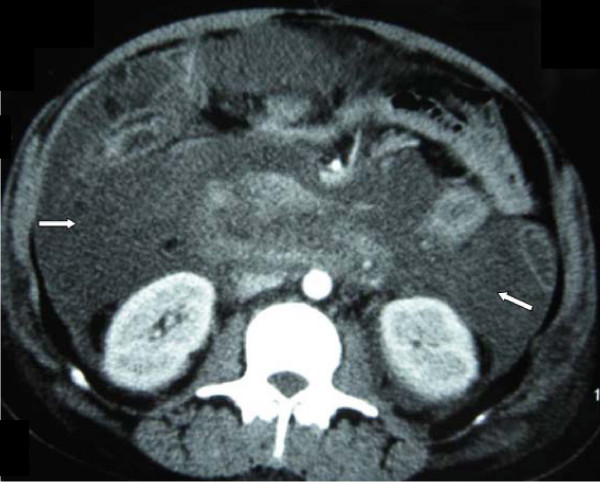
Contrast-enhanced computed tomography of pancreas when the patient was admitted. Enlarged necrotic pancreas and peripancreatic inflammation and fluid were shown in this picture. (arrows).
